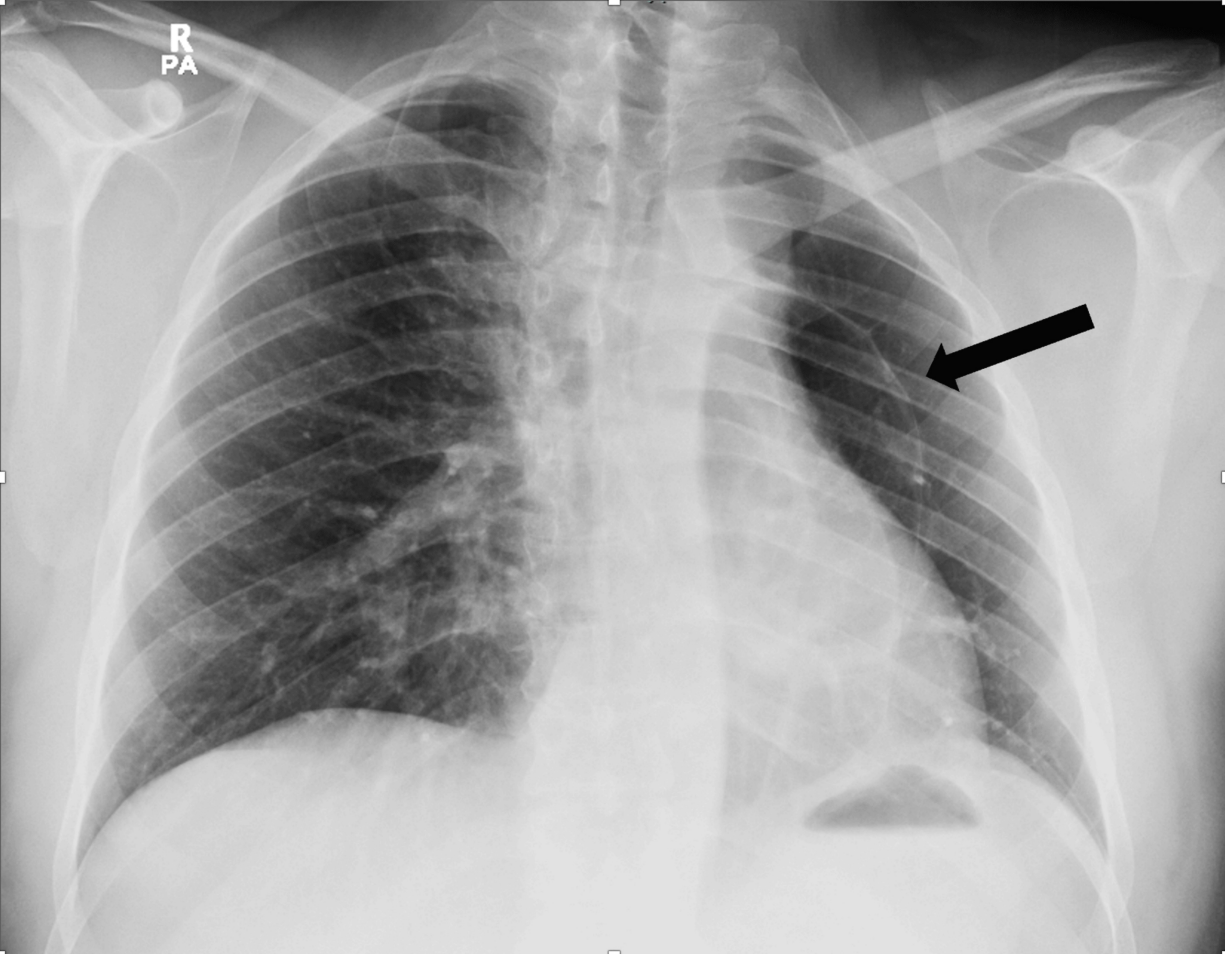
Chest X-ray shows reduced volume of the left lung with a cystic structure at the left lung hilum (arrow).
